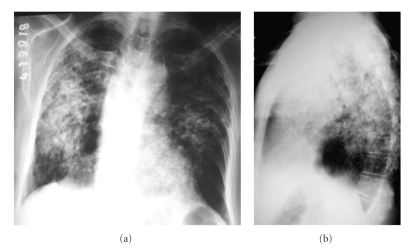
Chest radiographs in anteroposterior (a) and lateral incidence (b) showing hyperinflated lungs, with bilateral nonhomogeneous opacities, predominantly in the middle third of right lung.
